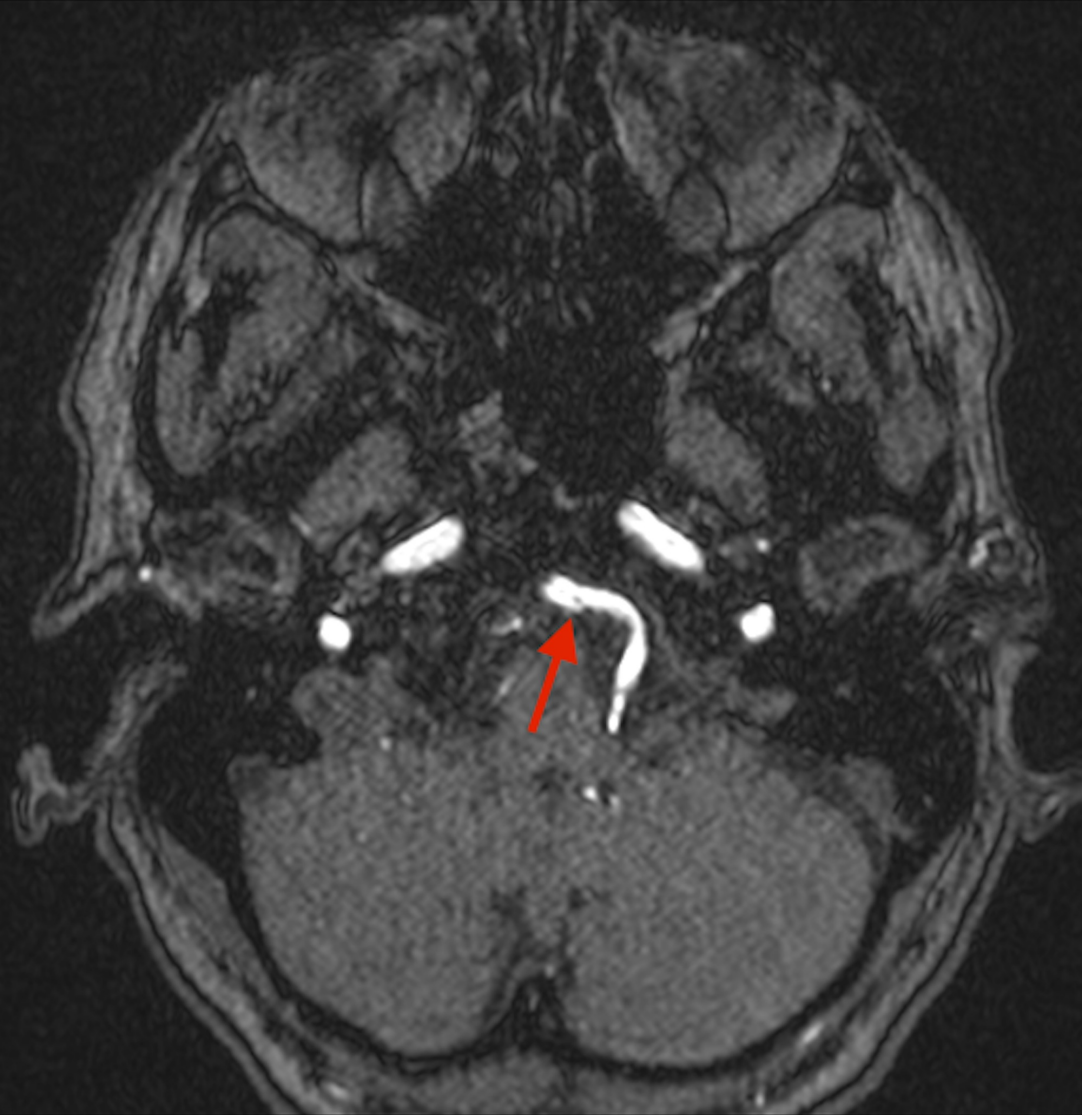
3 Dimension (D) Time of Flight (TOF) source data images reveal an intimal flap of the left V4 segment of the vertebral artery suggestive of an arterial dissection (red arrow) MRI: Magnetic Resonance Imaging
